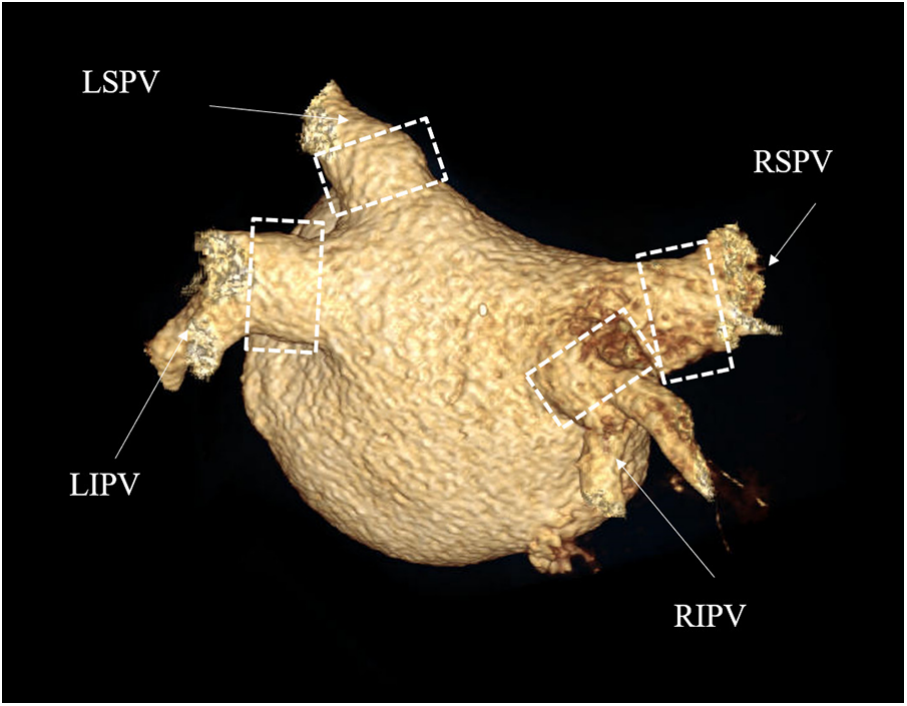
CTA of pulmonary veins. Postoperative 3D reconstruction images of the pulmonary veins. The four branches of the pulmonary veins were normal in size and direction, and no pulmonary vein stenosis occurred. The white dashed box is the location of the surgical ablation. RSPV, right superior pulmonary vein; RIPV, right inferior pulmonary vein; LSPV, left superior pulmonary vein; LIPV, left inferior pulmonary vein.
